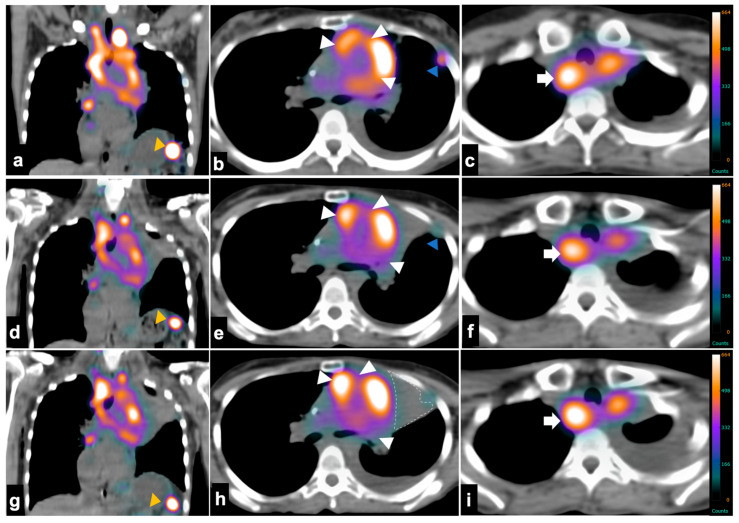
Coronal and axial fused single-photon emission tomography/computed tomography (SPECT/CT) after the first (a–c), second (d–f) and third (g–i) cycles of treatment with [^177^Lu]Lu-DOTA-MGS5. Imaging showed substantial stable disease over time (white arrowheads) with a slight response in small tumor lesions such as those in the gastrointestinal tract ((a,d,g)—yellow arrowheads) and in the left thoracic wall ((b,e,h)—blue arrowheads)—the same ones used for dosimetry studies. The retrotracheal mass (white arrow; (c,f,i)) appears to demonstrate constant uptake during the course of treatment.
